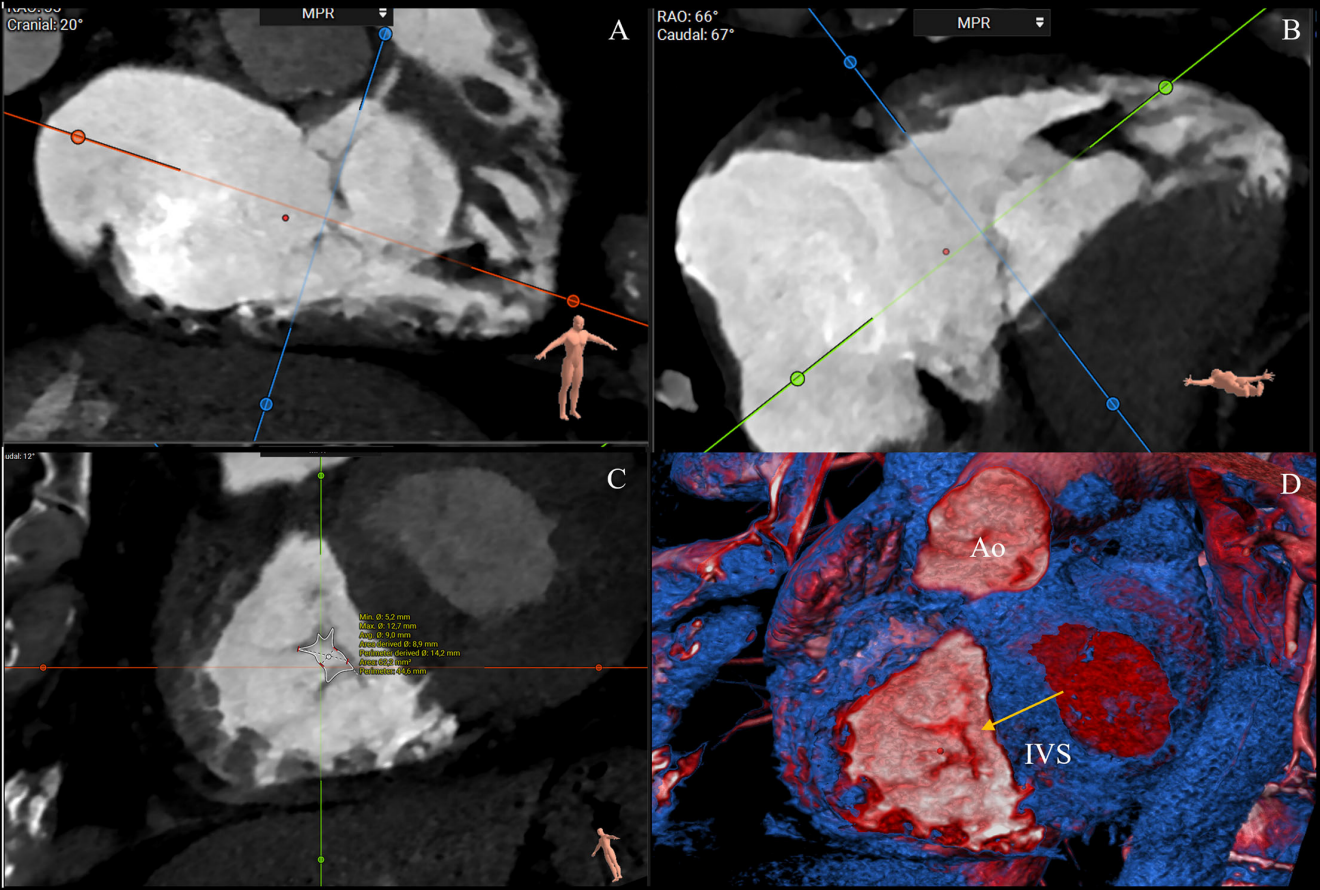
CT assessment of the anatomical regurgitant orifice area (AROA) using a manual approach. Multiplanar reconstruction is performed with the reformation planes aligned with the narrowest portion of the regurgitant orifice during mid-systole (A,B). Then, the contours of the AROA are manually traced on the reconstructed short axis (C) and tridimensional visualized in the volume rendering apical view (D, arrow) (3mensio Structural Heart; Pie Medical Imaging, Maastricht, The Netherlands). Ao, aorta; IVS, interventricular septum.
